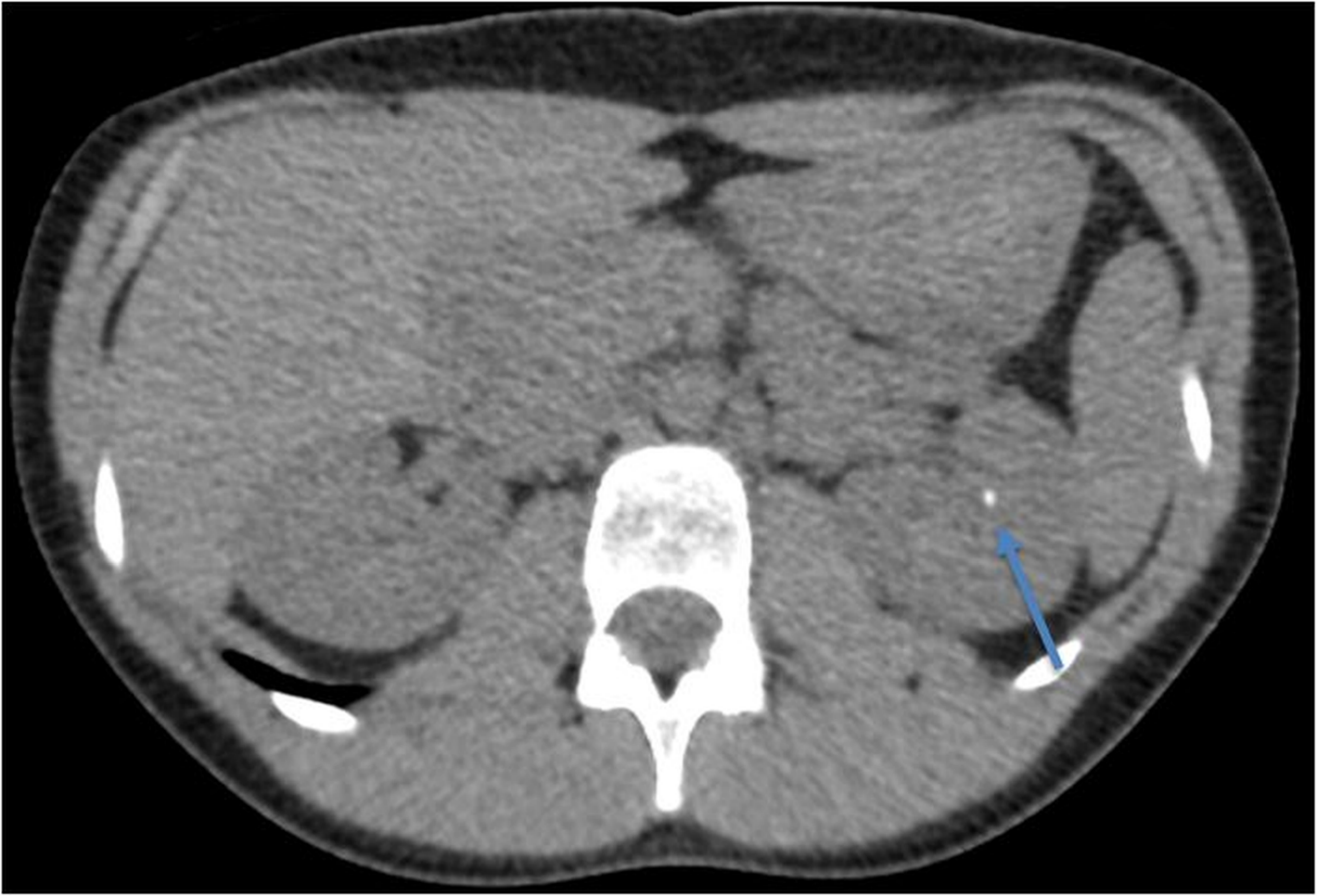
Transverse abdomen CT image (kidney stone protocol) of a 17-year-old female (53 kg) acquired at 2.3 mGy. Left kidney stone (arrow) and overall image quality scored optimal by four-fifths of the readers and sub-optimal by one-fifths of the readers. However, a diagnosis of kidney stone was unaffected by all readers. Median IQSC score was 3
